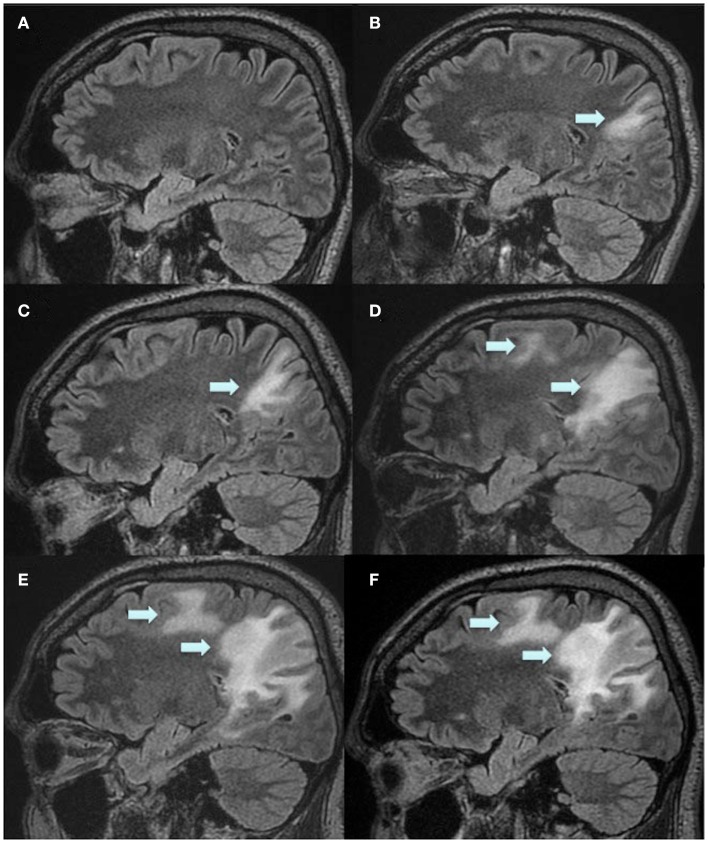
Magnetic resonance imaging scans during the course of PML and IRIS. Sagittal reconstructions through the right hemisphere from 3D Flair acquisition. Routine MRI 1 year before PML was diagnosed (A), when PML was diagnosed (B) and subsequent scans 2 weeks (C), 1.5 months (D), 2.5 months (E), and 3.5 months (F) after plasma exchange. Arrows indicate the PML lesions.
